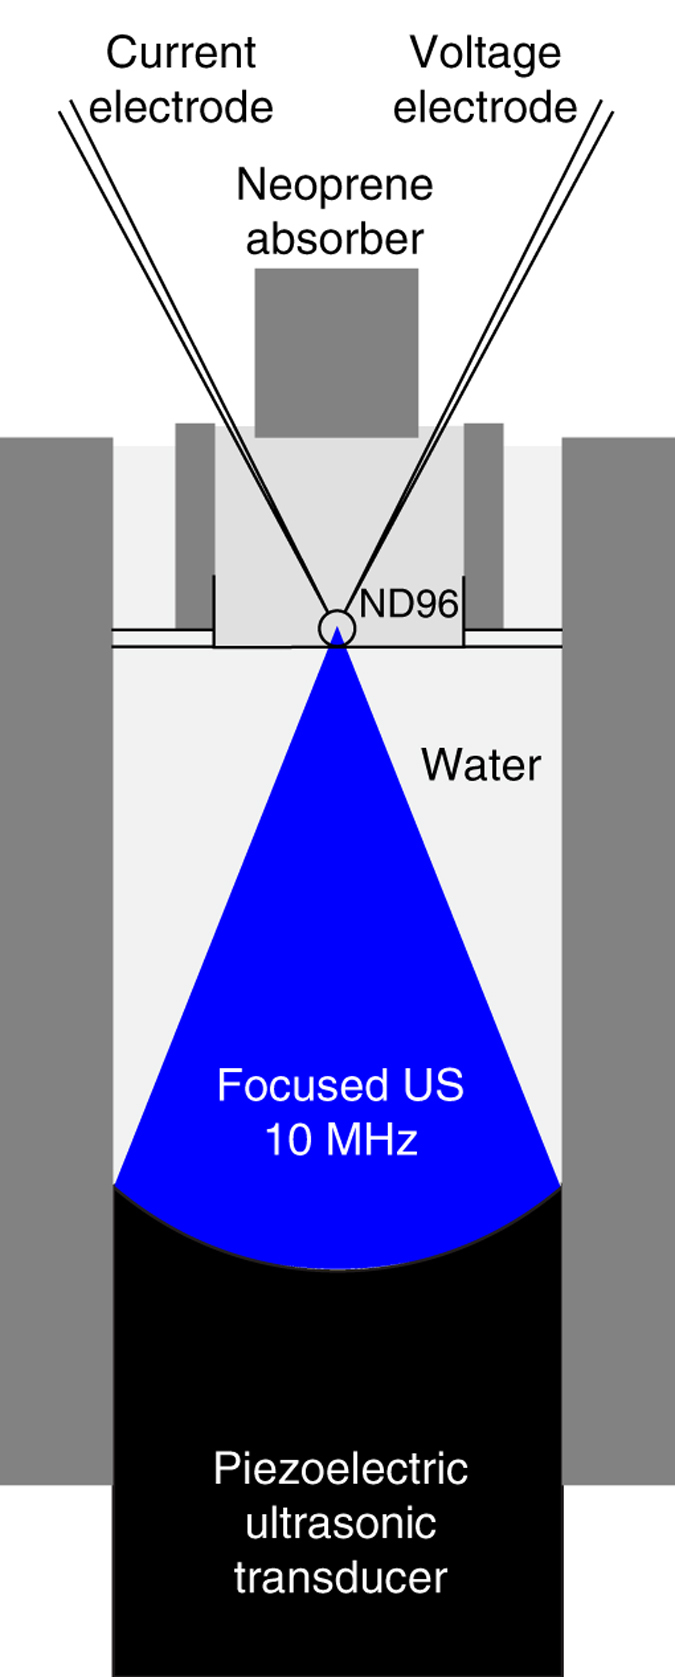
Measurement of currents flowing through ion channels in response to ultrasonic stimulation. A *Xenopus* oocyte expressing an ion channel of interest is placed in a recording bath in a chamber (see Methods), and subjected to an US pressure field generated by a focused US transducer placed below the chamber and submerged in deionized and degassed water. A neoprene absorber is immersed in the recording bath to prevent the formation of a standing wave in the chamber. Transmembrane currents are measured using the two-electrode voltage clamp.
